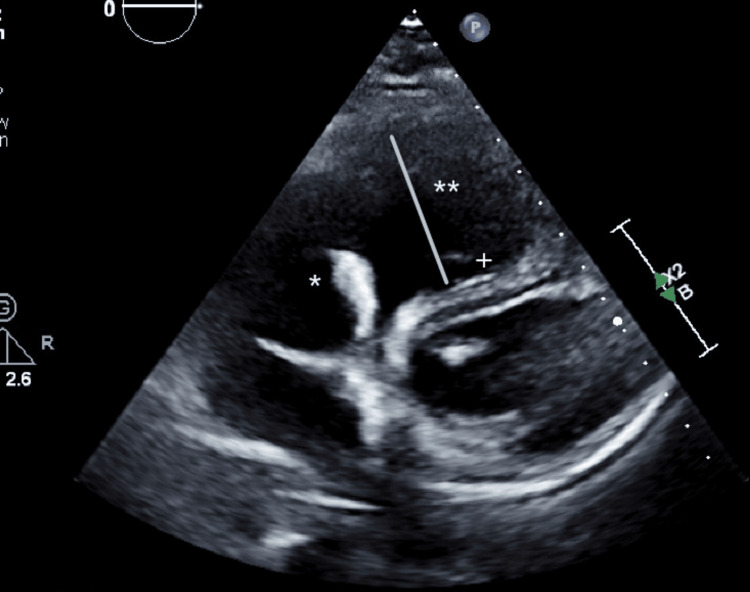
Transesophageal echocardiogram short-axis cut showing the abnormal structure (asterisk), the D-shaped right ventricle (double asterisks), and flattened septum (plus sign). The D-shaped right ventricle and flattened septum are findings found in right ventricle overload
